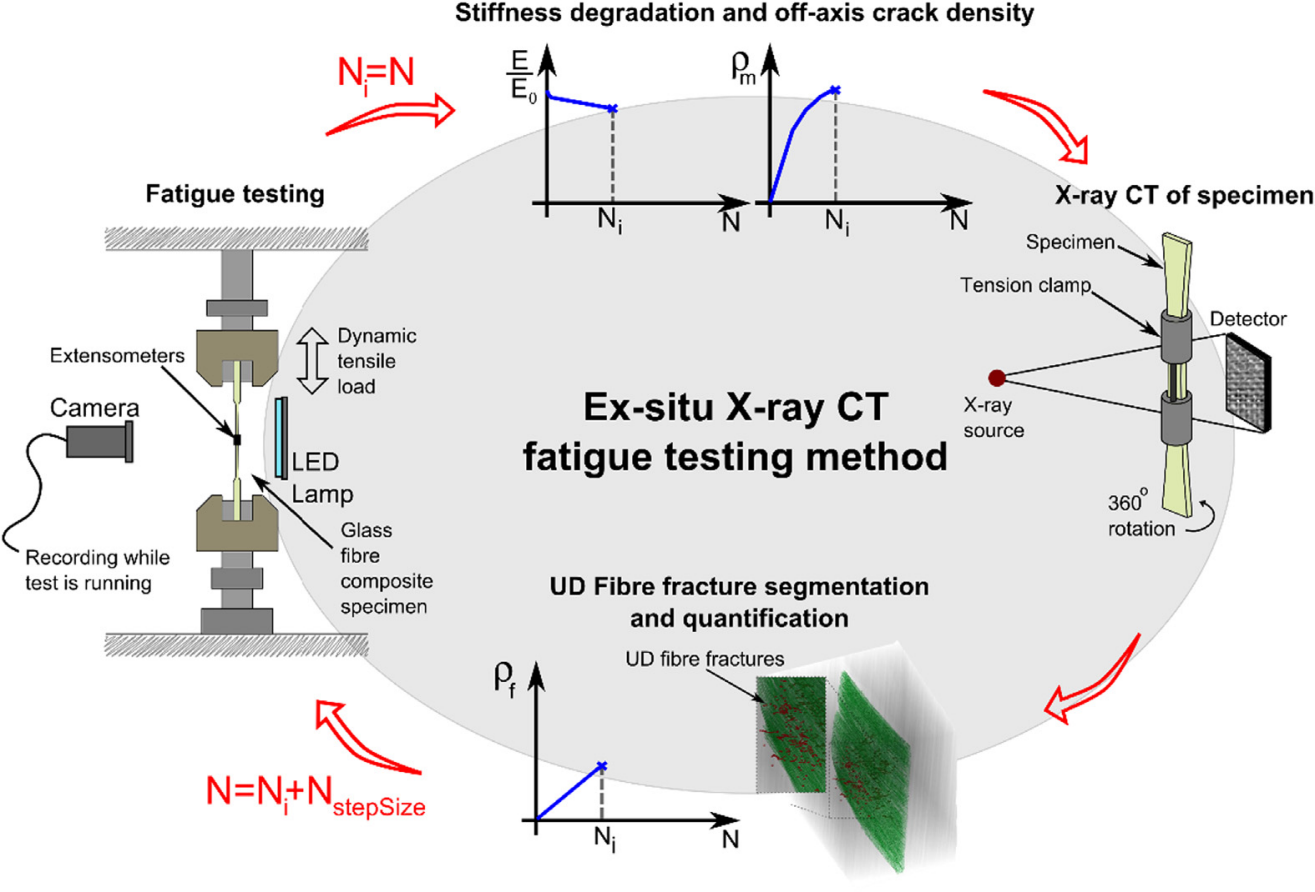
Overall idea behind ex-situ X-ray CT fatigue testing approach supplemented by TWLI and tension clamp.
